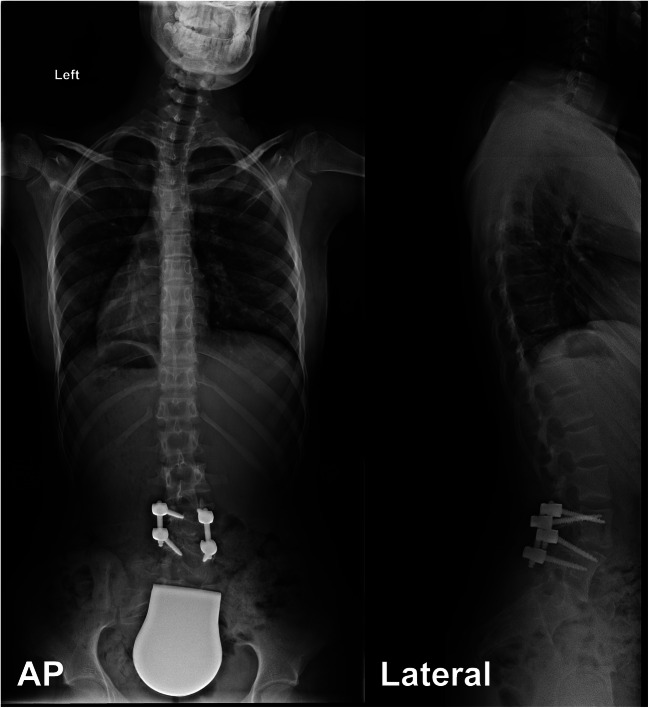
Postoperative imaging. Total spine x-ray in ap and lateral view (standing)
